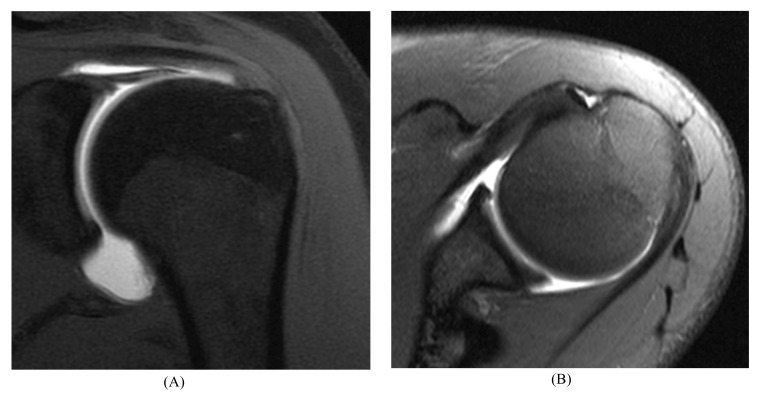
Normal labrum. 14 year-old male athlete with an unequivocally normal labrum. (A) Coronal and (B) axial fat-suppressed T1-weighted MR arthrogram images show normal low signal intensity fibrocartilage; no contrast material extends into the labrum or into the chondrolabral junction.
